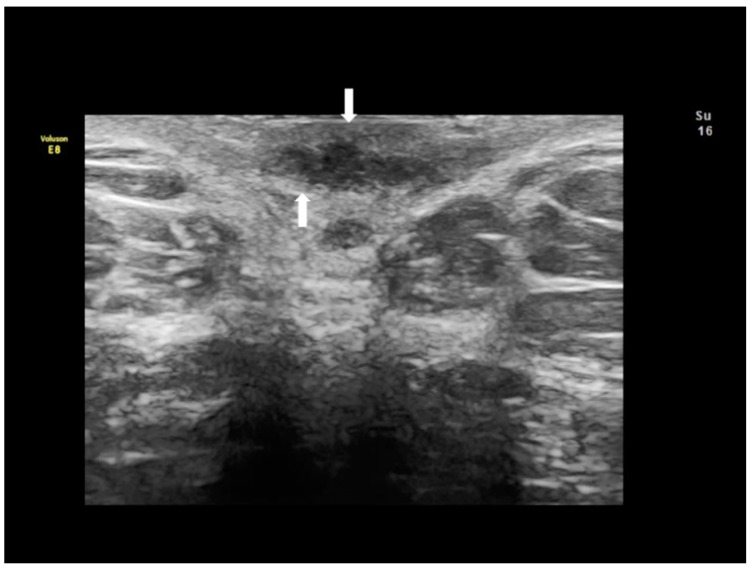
The ultrasonographic appearance of a Villar’s nodule (see white arrows) in a woman without previous abdominal surgery.
